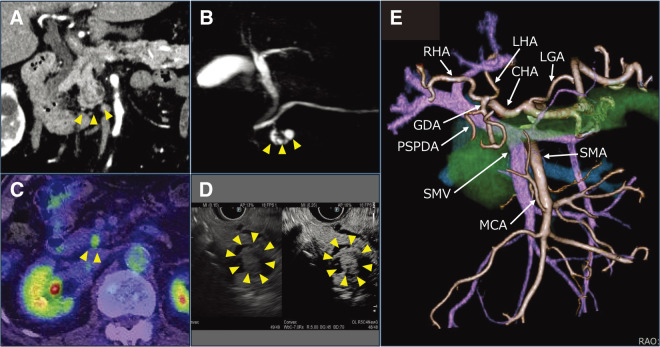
Preoperative imaging studies. (A) Contrast-enhanced CT image. (B) Magnetic resonance cholangiopancreatography imaging. (C) Positron emission tomography/CT image. (D) Endoscopic ultrasonography image. (E) Three-dimensional image of the main vessels and pancreas. Increased fluorodeoxyglucose uptake in the pancreatic head and enhanced mural nodule detected via endoscopic ultrasonography raised suspicion of malignancy. CT, computed tomography; RHA, right hepatic artery; LHA, left hepatic artery; CHA, common hepatic artery; LGA, left gastric artery; SMA, superior mesenteric artery; MCA, middle colic artery; SMV, superior mesenteric vein; PSPDA, posterior superior pancreaticoduodenal artery; GDA, gastroduodenal artery
